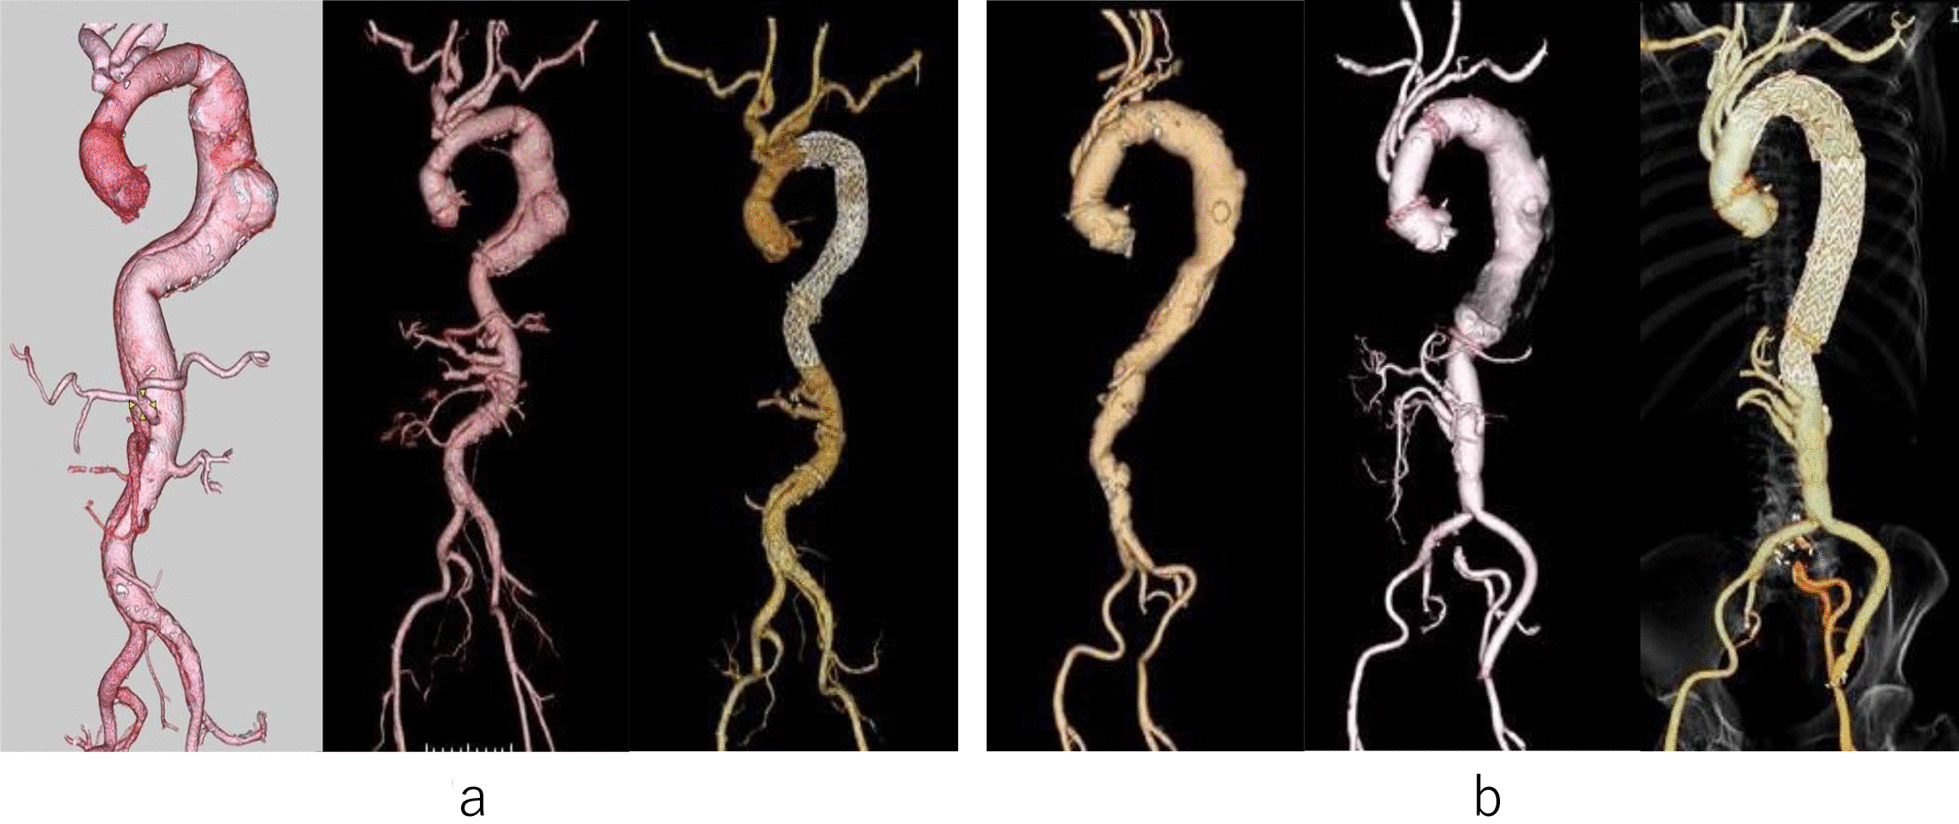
3D-CTA images of case 2 and 3. a The abdominal aorta of case 2 during the preoperative period and after the primary open surgery and hybrid repair; b the abdominal aorta of case 3 during the preoperative period and after the primary open surgery and hybrid repair
